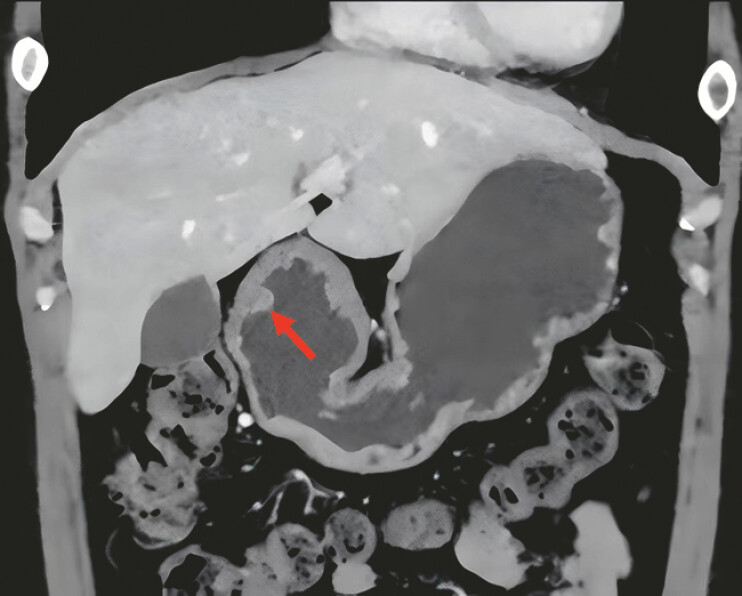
Enhanced computed tomography revealed the presence of a high density lesion in the gastric antrum (arrows).
